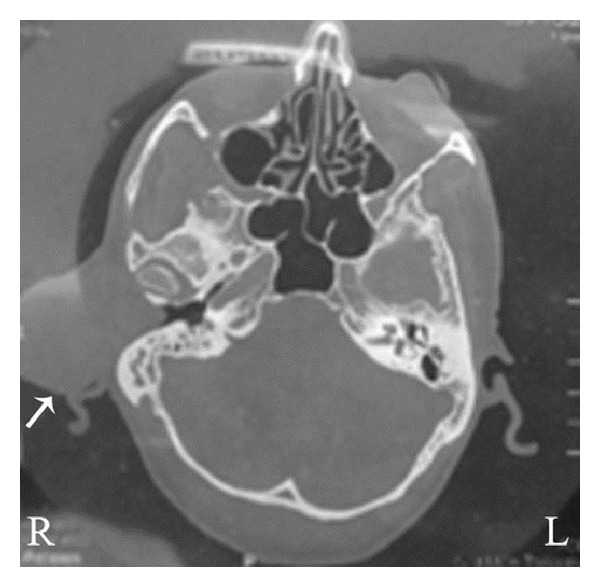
CT image shows the axial section of both temporal bones. The arrow indicates the tumor mass situated anterior to the right pinna involving outer part of the right external auditory canal.
